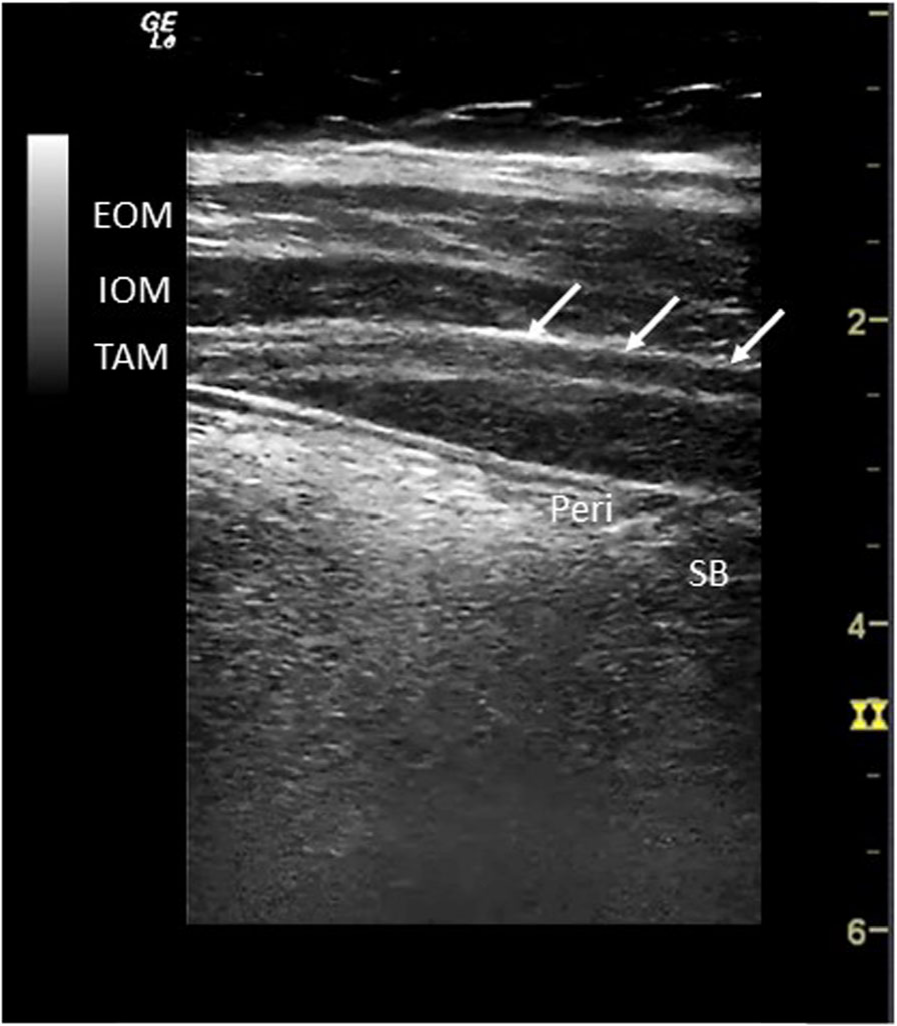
Typical sonogram of the abdominal wall in the lateral TAP position. Arrows indicate the TAP. EOM = external oblique muscle, IOM = internal oblique muscle, TAM transverse abdominal muscle, Peri = peritoneum, SB = small bowel
